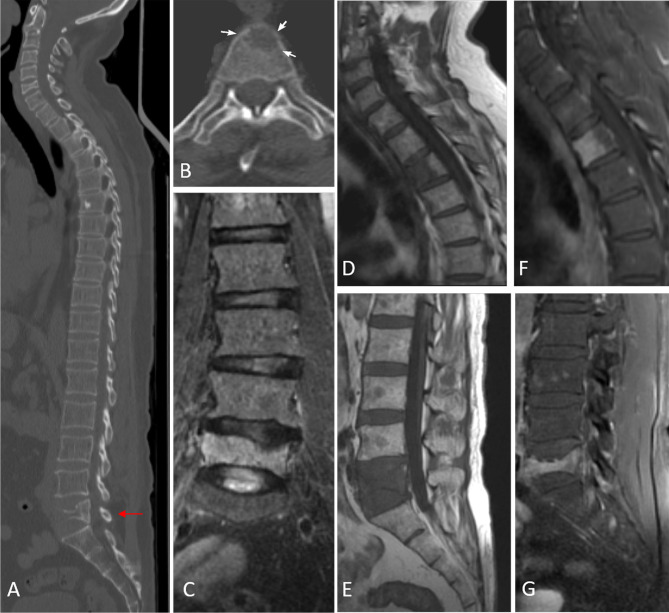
Image (A) sagittal CT image in bone window demonstrating vertebral compression fracture with >75% height reduction (arrow). Image (B) shows a lytic lesion involving the C7 vertebral body. MRI images confirm pathological L5 vertebral fracture with the concave posterior bulge of the vertebra, STIR hyperintensity seen in coronal STIR image of the lumbar spine [image (C)], T1 hypointensity [image (E)], and post-contrast enhancement [image (G)]. The lytic cervical vertebral lesion seen on CT in image (B), is well appreciated in images (D, F). Additionally, MRI reveals a variegated pattern with multiple punctate foci of altered signal intensity involving the marrow.
